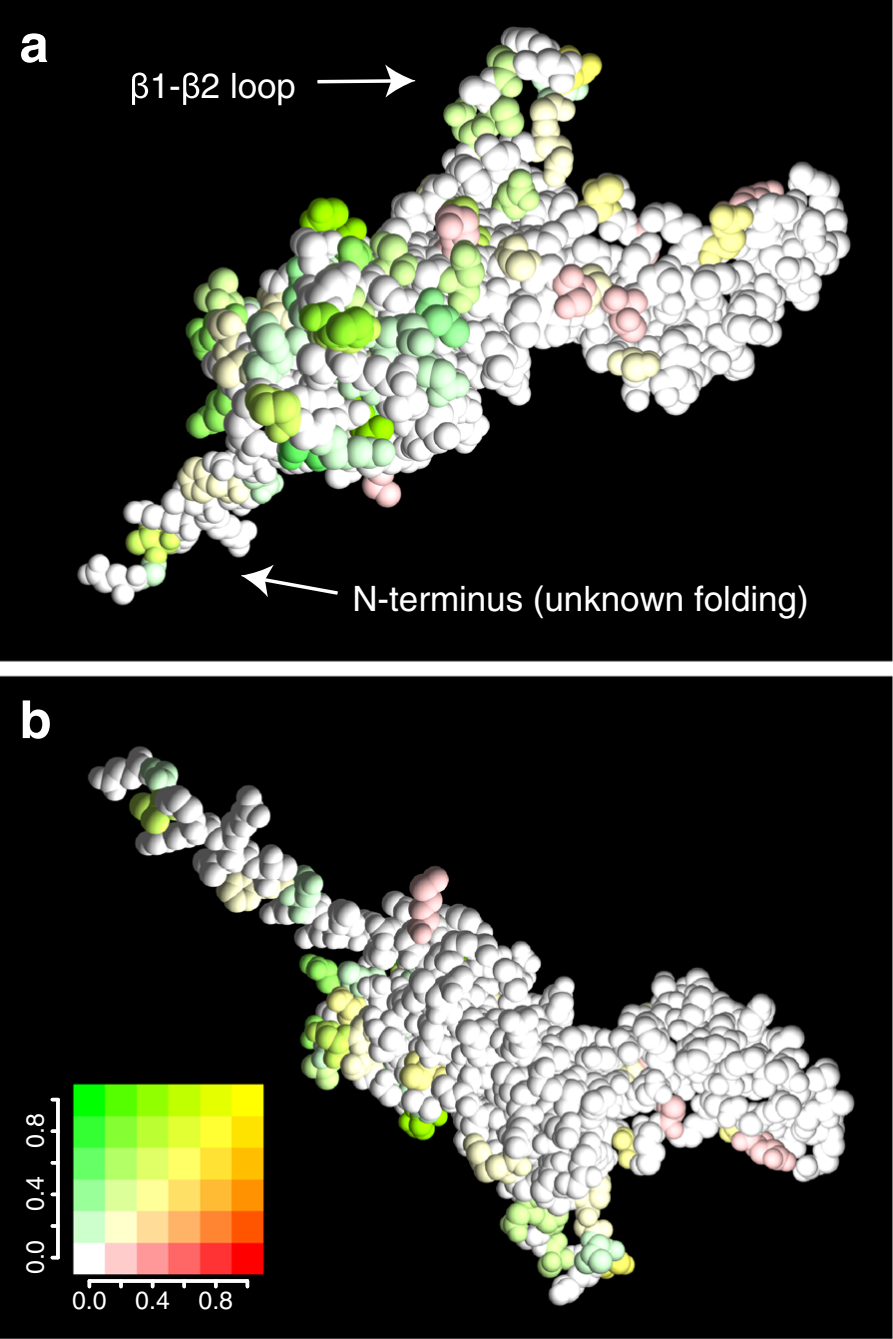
Mapping variable amino acid residues on the PsbO structure. a View from thylakoid lumen, (b) view from PSII. Differences between isoforms (in green) are merged with differences between species (in red); the frequency of particular differences on each position is indicated by colour gradient. The homology model of the *Solanum tuberosum* PsbO2 based on the X-ray structure of cyanobacterial PsbO [PDB:3ARC] [5] was constructed using Swiss-Model program [38]; the first 13 N-terminal amino acids were not present in the template structure, so they were pasted in the model without attempts to show any folding
